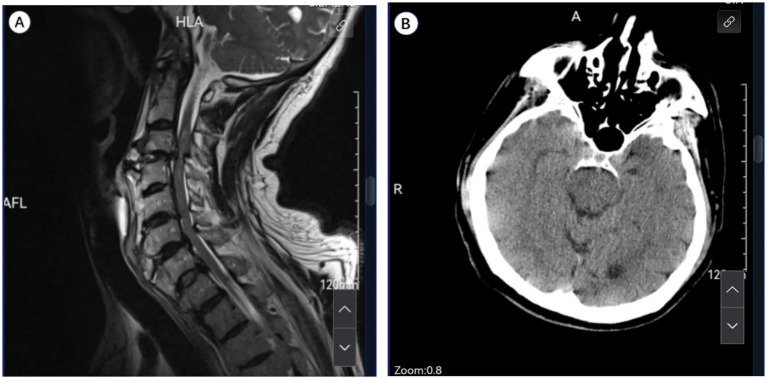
(A) Sagittal T2-weighted cervical spine MRI post-injury demonstrates spinal cord injury at C3-C4. (B) Initial emergency cranial CT reveals no intracranial abnormalities.
